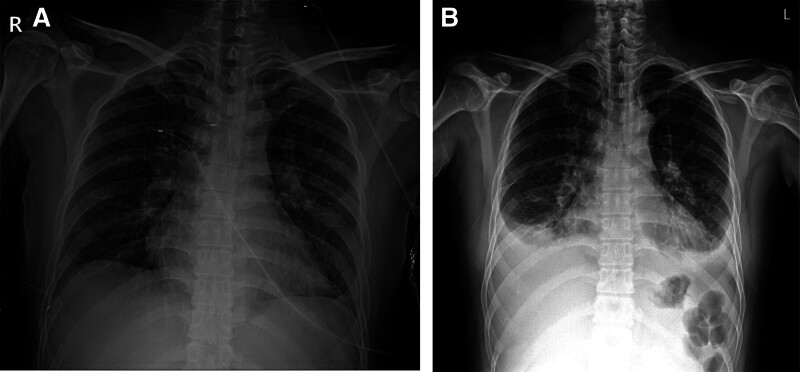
(A) Initial chest radiography. (B) Chest radiography at the time of patient disorientation.
